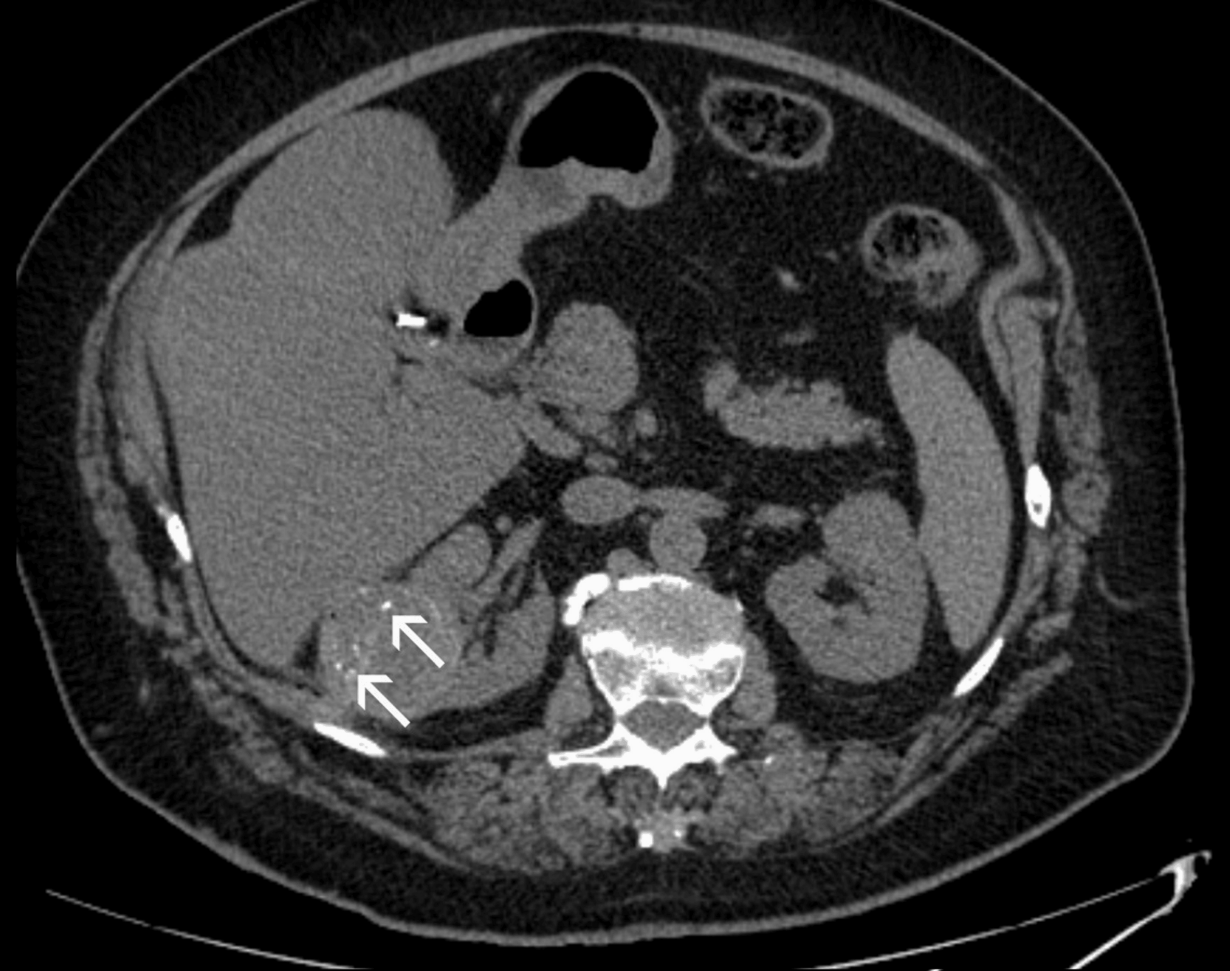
Unenhanced axial CT image immediately post ablation. No apparent complication following the procedure. Hyperdense intratumoral foci (arrows) are attributed to the embolic material (radiopaque microspheres) of TAE four weeks earlier.
